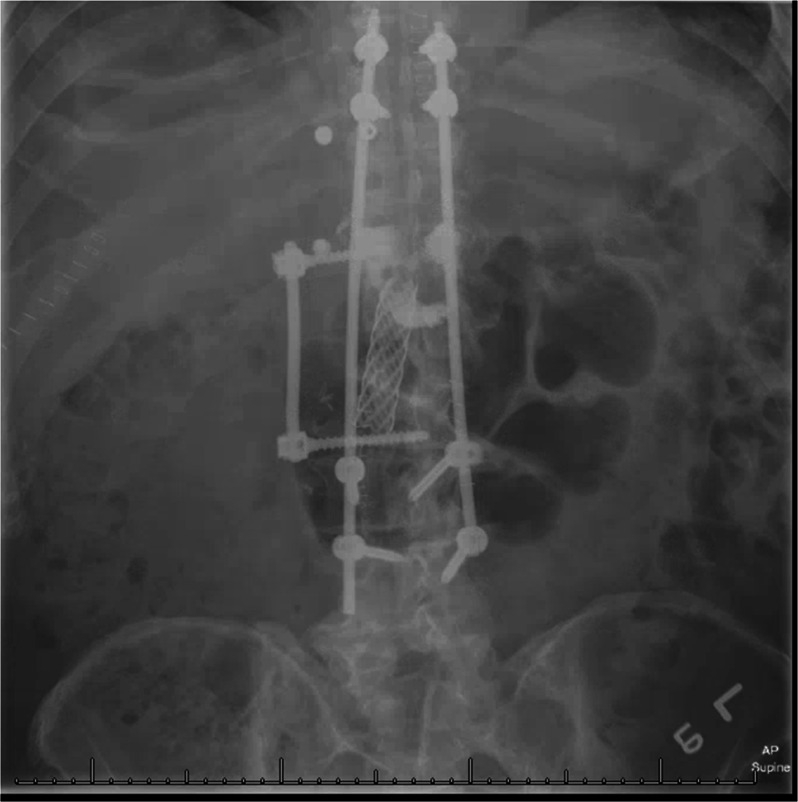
Upright anteroposterior radiograph of the thoracolumbar spine following an anterior corpectomy from T12-L2 and placement of an intervertebral biomechanical implant and stabilization with posterior T9-L4 instrumentation and fusion
